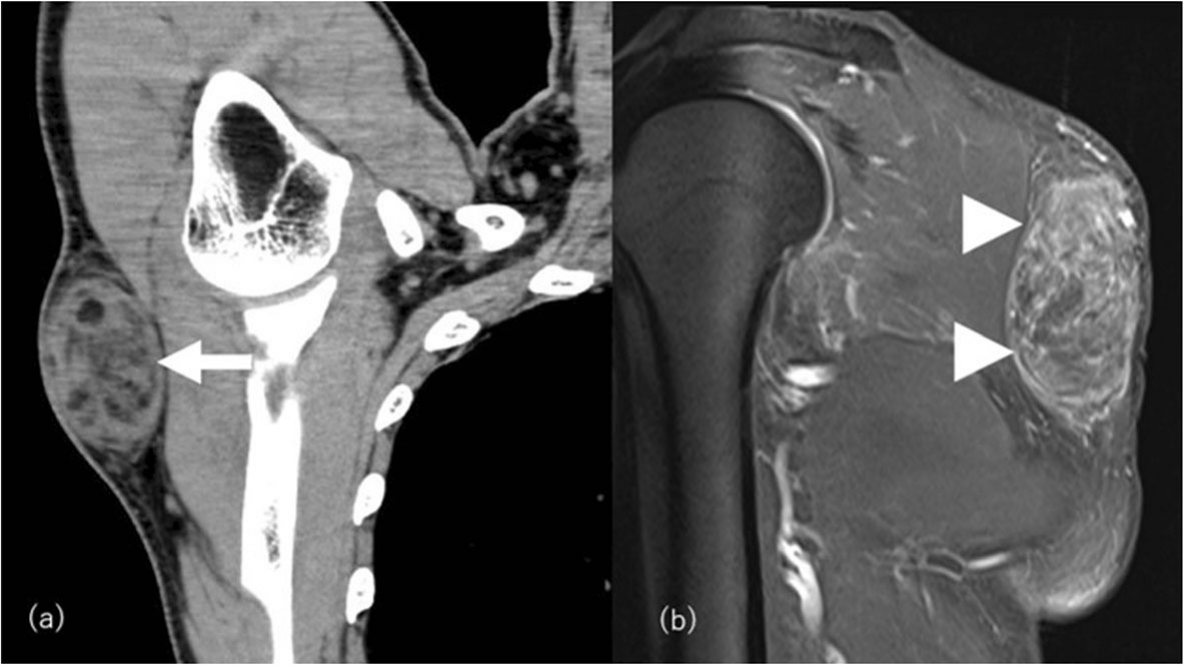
Atypical lipomatous tumor in a 62-year-old male with a painless, firm, and mobile mass gradually increasing in the right back. a Coronal CT image shows a fat-containing inhomogeneous density mass (arrow). b On post-contrast fat-saturated T1-weighted image, the non-fatty lesion shows moderate enhancement (arrowheads). Surgically diagnosed as ALT with abundant fat necrosis
